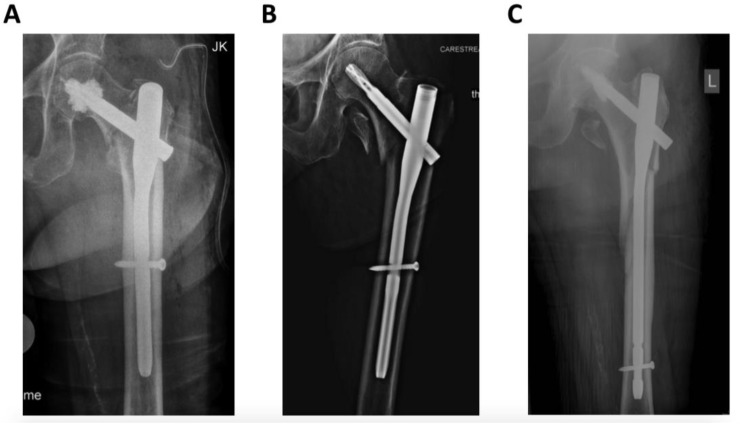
Radiographic images of different trochanteric fracture patterns according to the revised AO/OTA Classification. (A) = 31A1; (B) = 31A2; (C) = 31A3.
